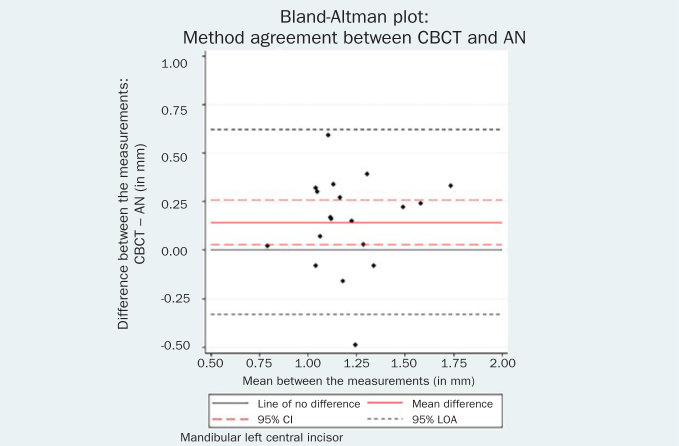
Bland-Altman plot CBCT vs AN 31.
